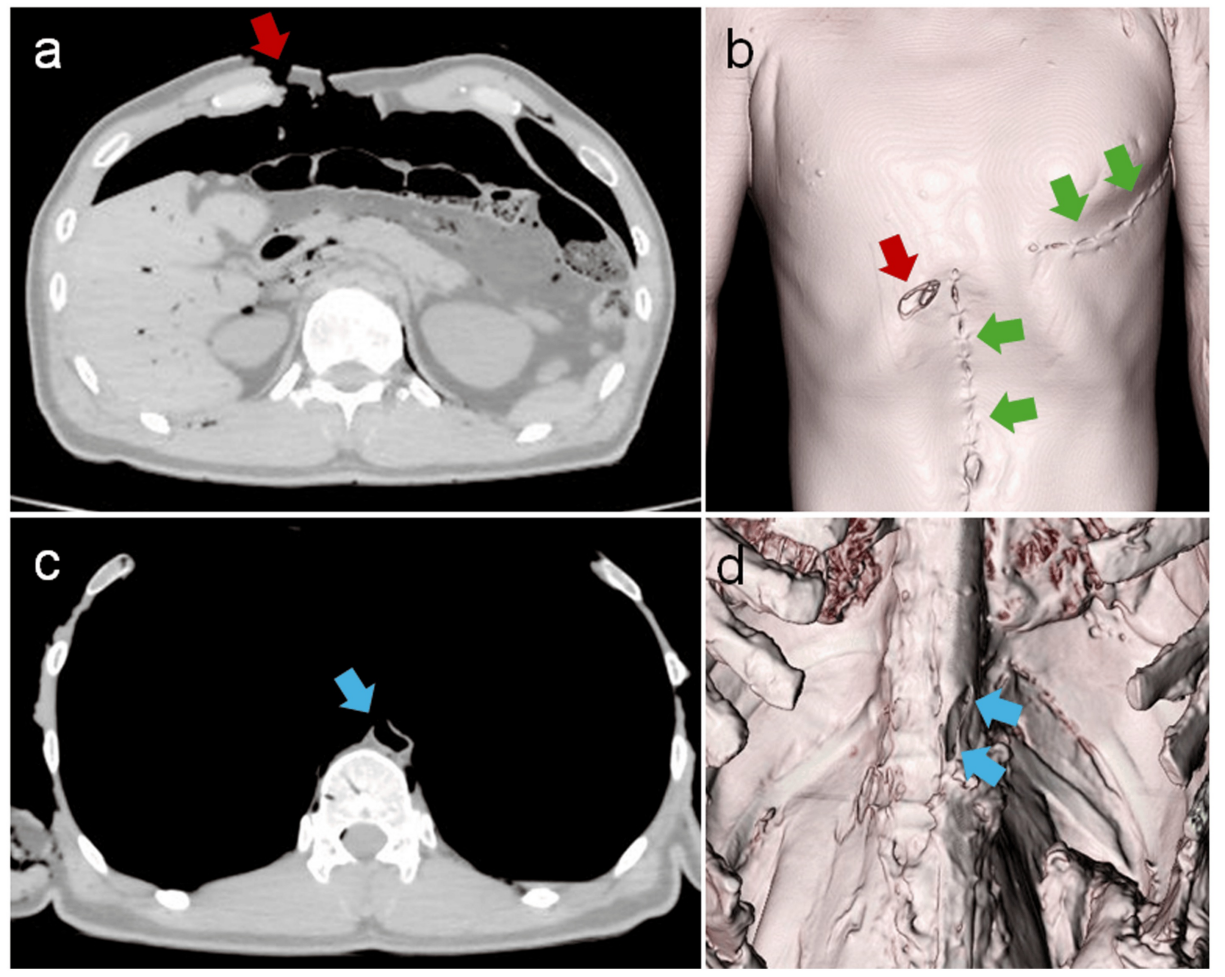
Pre-autopsy and intra-autopsy CT images. a. 2D horizontal cross-sectional image of the chest and abdomen from pre-autopsy CT. A stab wound in the right hypochondriac area, indicated by the red arrow, and free air in the peritoneal cavity were observed. b. 3D volume-rendered image from pre-autopsy CT. The stab wound in the right hypochondriac area is visible, indicated by the red arrow. The green arrows indicate treatment scars from the hospital where emergency care was administered. c. 2D horizontal cross-sectional image of the chest and abdomen from intra-autopsy CT. The severed section of the abdominal aorta is visible, indicated by the blue arrow. d. 3D volume-rendered image from intra-autopsy CT. The severed section of the abdominal aorta is visible, indicated by the blue arrows.
